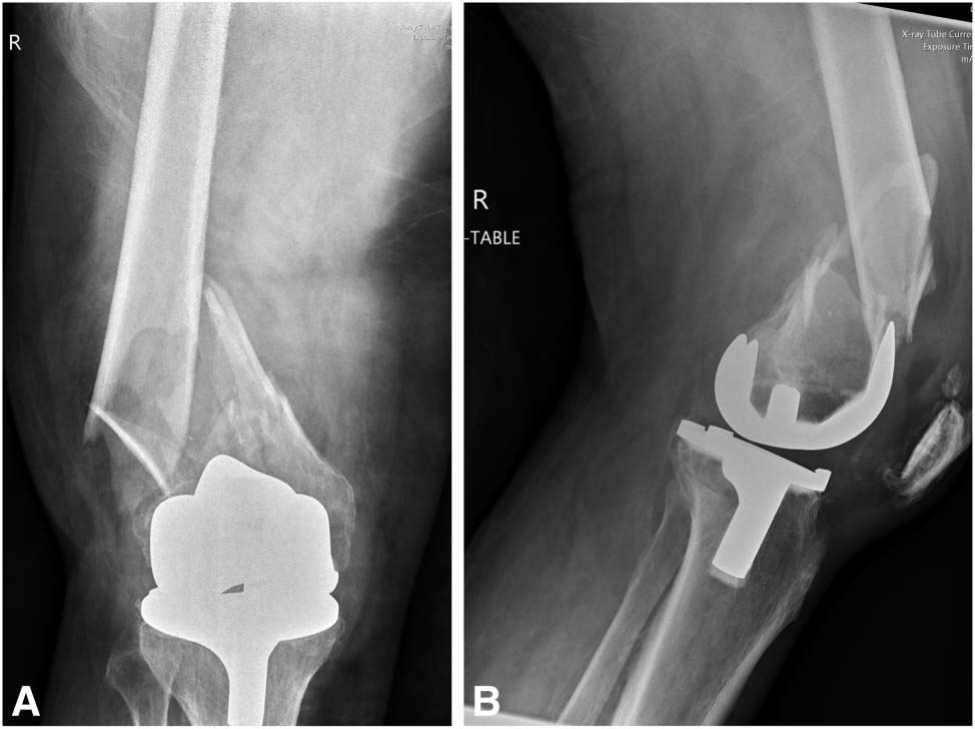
A, AP and (B) lateral radiographs of the patient's right knee immediately upon presenting to the emergency department demonstrate a comminuted and displaced periprosthetic supracondylar distal femur fracture.
